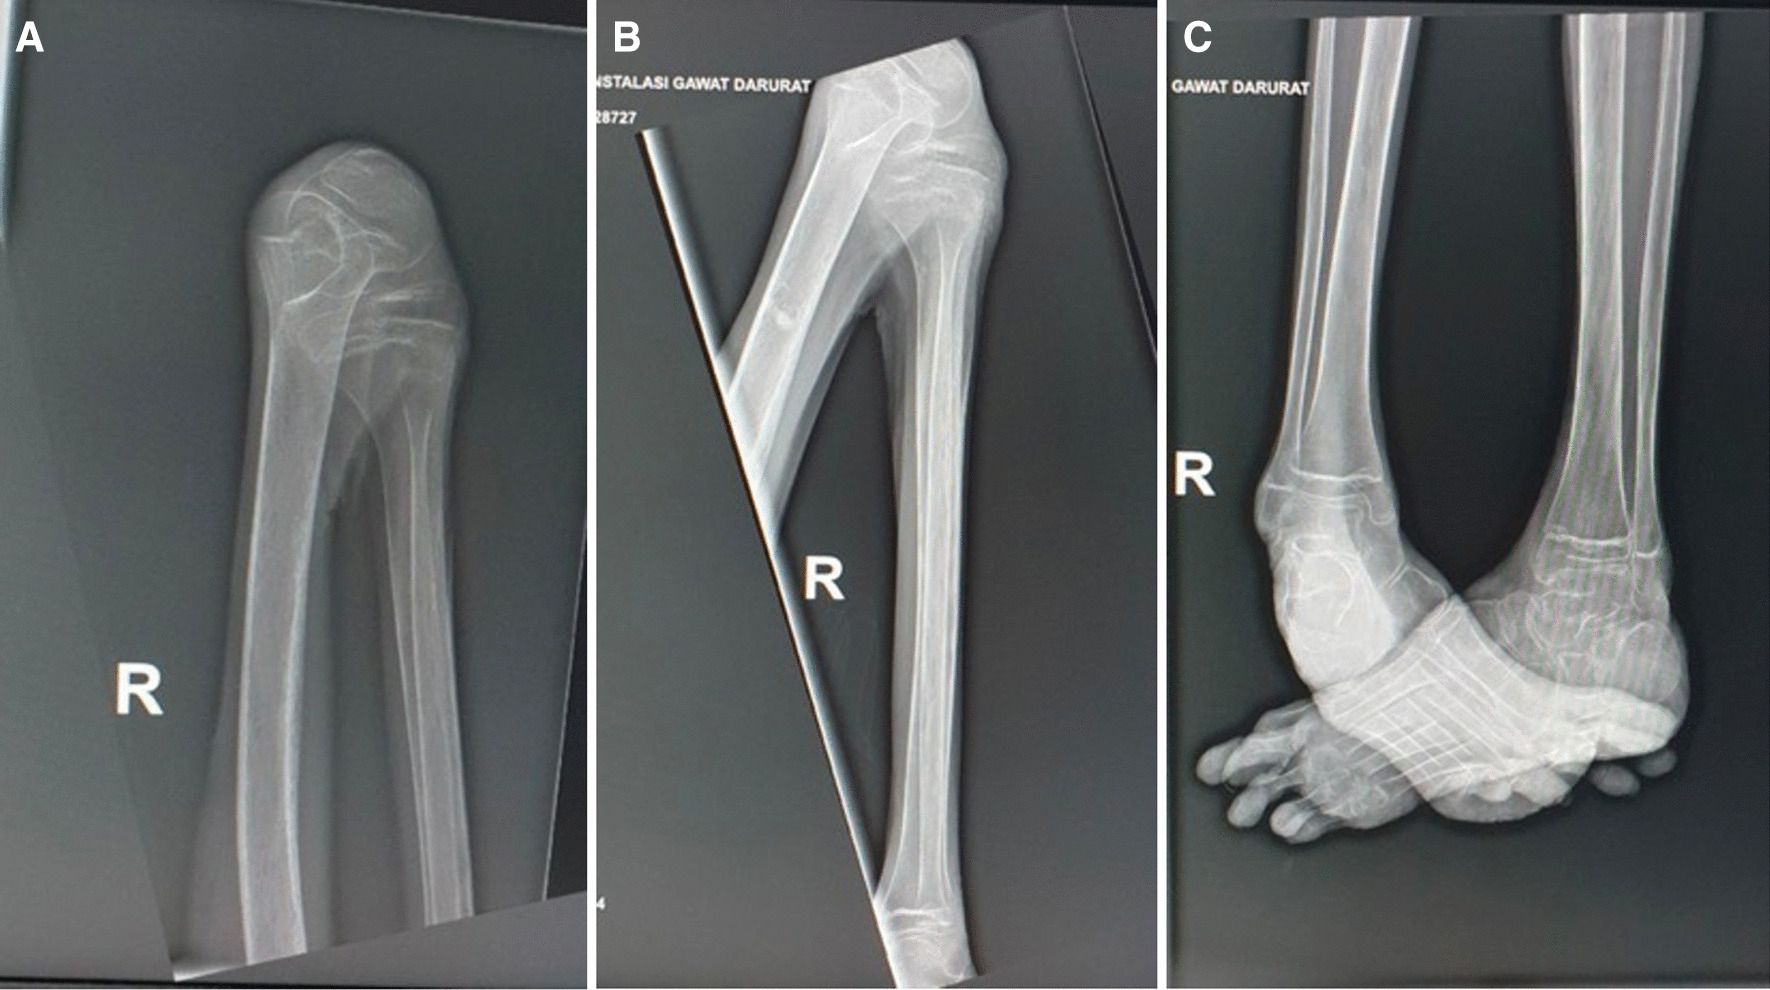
Lateral plain radiographs of femur (A, B) and AP plain radiographs of cruris bilateral (C), showing decreased density and porotic bone, flexion deformity of genu and ankle, and bilateral ankle inversion without fracture or bone destruction
